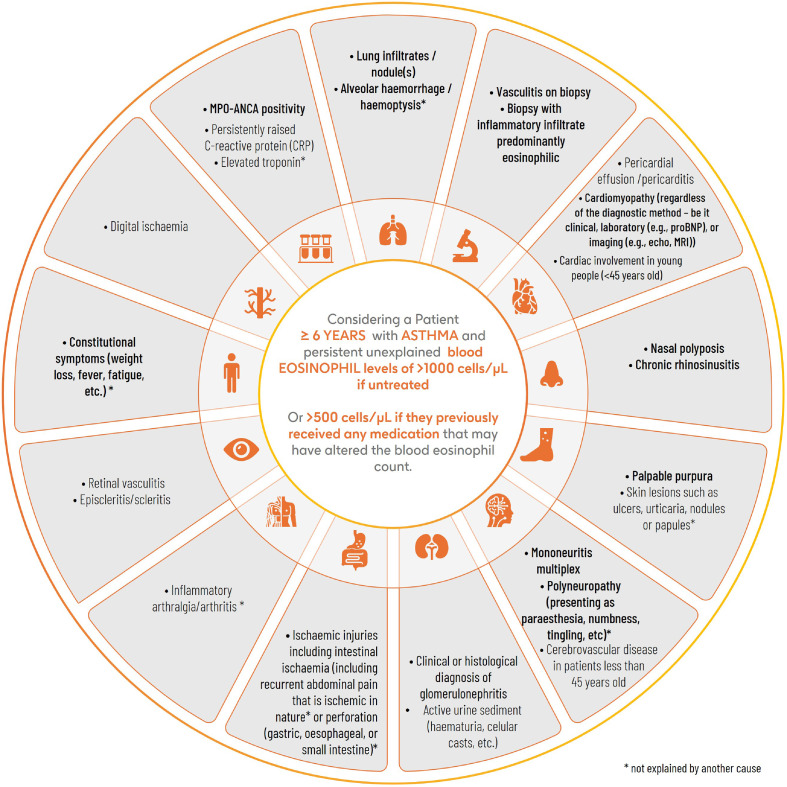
List of agreed red flags for EGPA suspicion. Items in bold correspond to the 12 most relevant red flags based on both clinical relevance and frequency of observation. Clinical relevance is understood as their practical importance or applicability to real-world patient care to influence clinical decision-making, and frequency of observation according to the panellist’s clinical experience. EGPA, eosinophilic granulomatosis with polyangiitis; MPO-ANCA, myeloperoxidase–antineutrophil cytoplasmic antibodies; MRI, magnetic resonance imaging; N, number of panellists; proBNP, B-type natriuretic pro-peptide.
